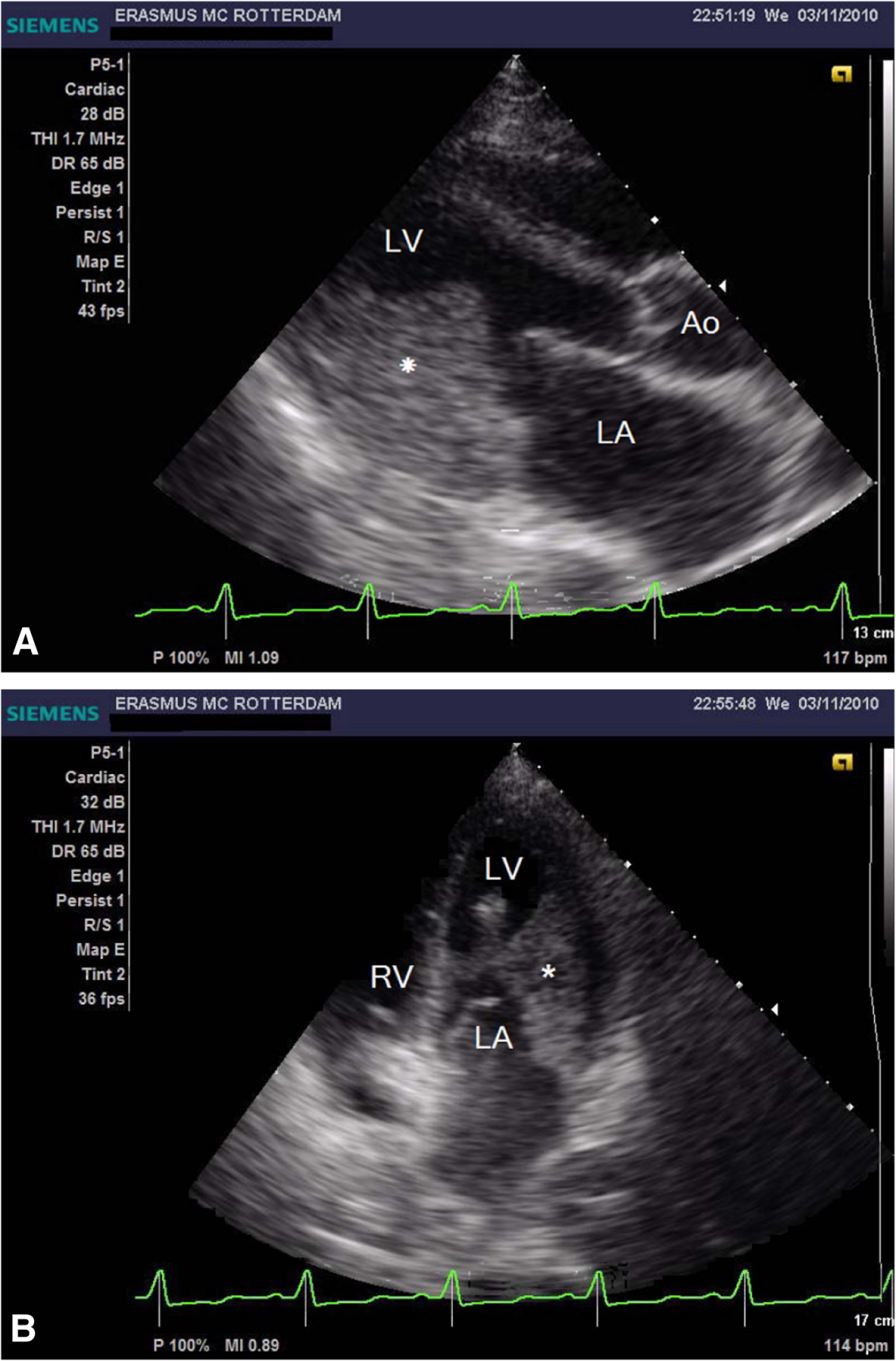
A and B, Echocardiographic parasternal long axis view and four-chamber view at presentation, showing big thrombotic mass with the base at the posterior wall and infiltration of the left ventricular wall.
